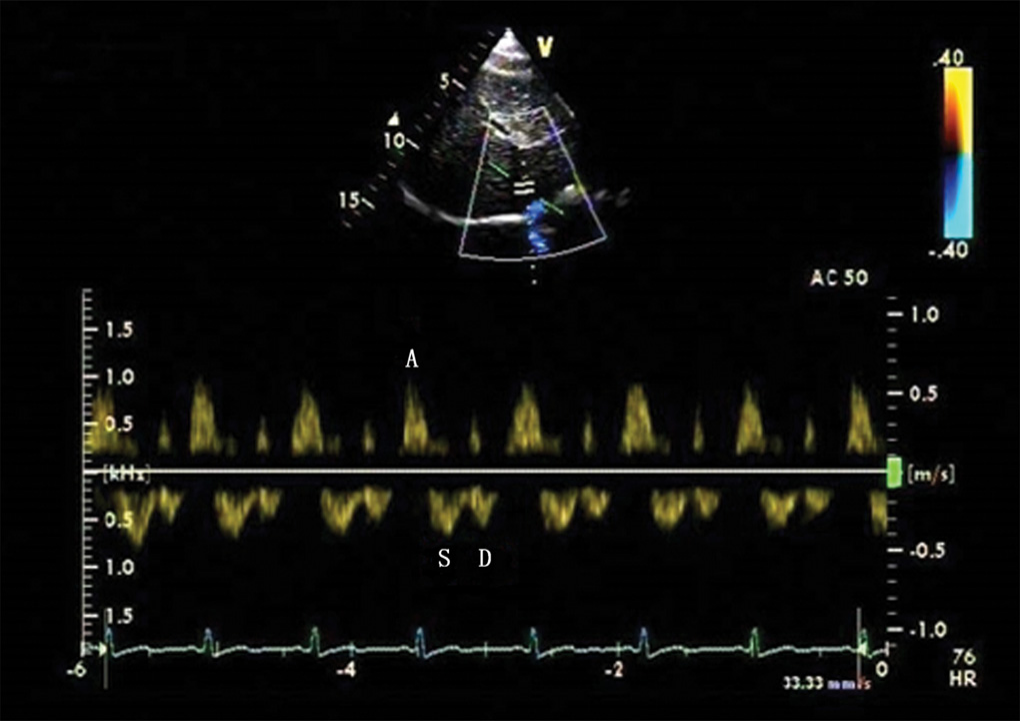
Four-phasic Doppler flow spectra of the MHV in the pulmonary hypertension (PH) group. Doppler spectra of MHV obtained from a patient with ventricular septal defect with the MPAP of 80 mmHg. The four-phasic waveform includes S wave, D wave, A wave and ventricular reversal (V wave) waves. Compared with the control group, the spectra had a higher value of the peak flow velocity and the velocity time integral (VTI) of A wave, and a lower value of peak flow velocity and VTI of S wave and D wave.
